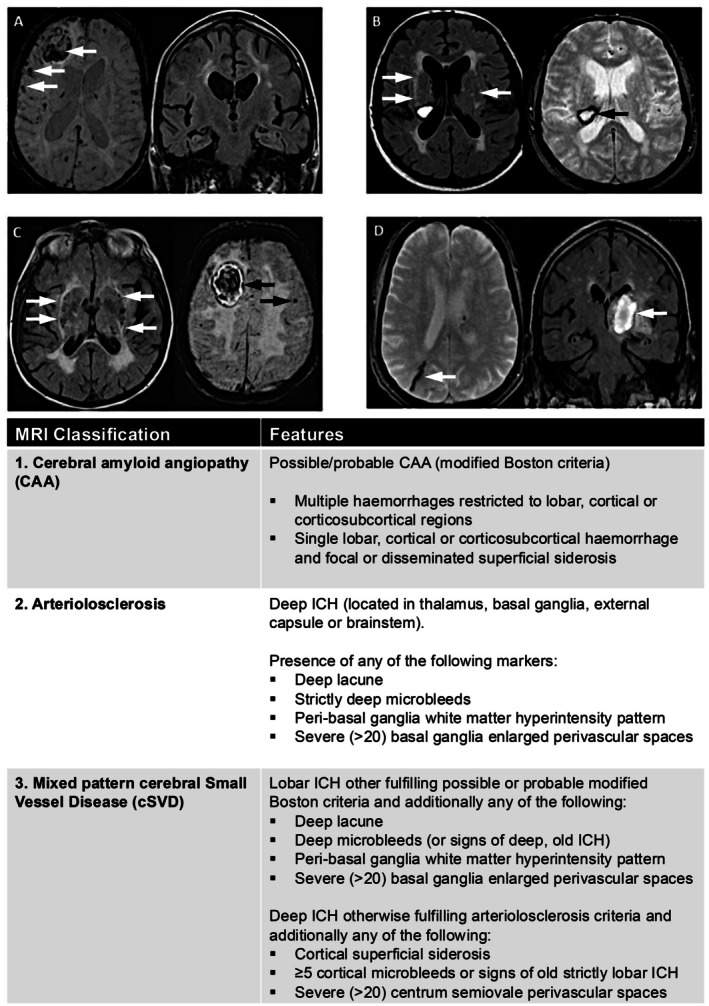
MRI‐based classification of ICH. Images showing (A) probable CAA: lobar ICH with lobar CMB (both arrowed), no deep pathology; (B) arteriolosclerosis: no lobar CMB/cSS, deep ICH (arrowed) and peri‐BG WMH (arrowed); (C) mixed pattern SVD: lobar ICH and lobar CMB, deep and peri‐BG WMH (arrowed) and BG‐PVS; (D) mixed pattern SVD: focal cSS (arrowed), deep ICH located in the BG (arrowed). BG indicates basal ganglia; BG‐PVS; basal ganglia perivascular space; CAA, cerebral amyloid angiopathy; CMB, cerebral microbleed; CS‐PVS, centrum semiovale perivascular space; cSS, cortical superficial siderosis; ICH, intracerebral hemorrhage; MRI, magnetic resonance imaging; SVD, cerebral small vessel disease; and WMH, white matter hyperintensity.
